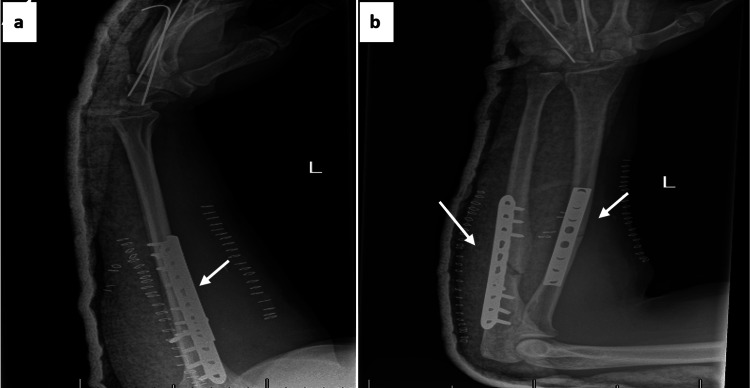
Postoperative radiographs: (a) lateral view showing stable fixation of forearm fractures, (b) anteroposterior view confirming anatomical reduction and implant position
